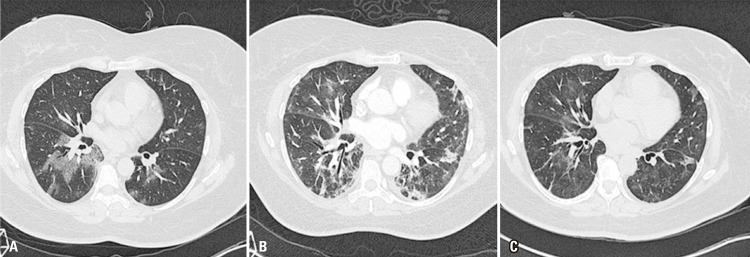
High-resolution CT scans showing progression of patient with severe pneumonia and COVID-19 (High resolution computed tomography – axial sections): (A) computed tomography at hospitalar admission, with bilateral patchy ground-glass opacities; (B) worsening of tomographic pattern at follow-up CT performed 17 days later, with emergence of parenchymal bands, reticular and some perilobular opacities, mild architectural distortion and irregular bronchial walls; (C) long-term CT follow-up (45 days after baseline CT) showing significant reduction of pulmonary abnormalities
